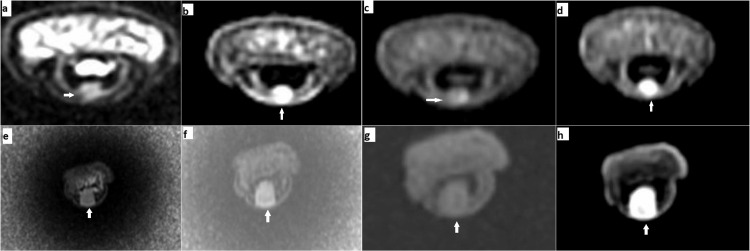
MRI findings of the index and little fingers (a,b,c,d - index finger; e,f,g,h - little finger). Axial T2-weighted image shows an iso-intense lesion in the distal phalanx of the index finger (a) and distal phalanx of the little finger (e). Short inversion time inversion recovery (STIR) images suggest the lesion was more conspicuous (b,f). Hypo-intense on T1-weighted fat-saturated image (c,g). T1 post-gadolinium fat saturation images show avid homogenous post-contrast enhancement (d,h).
